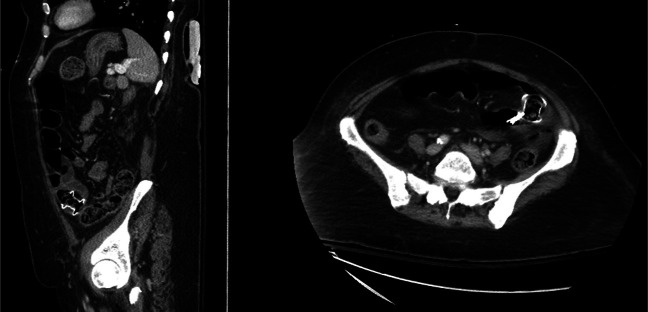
Computed tomography of the abdomen showing lumen apposing stent placement.
